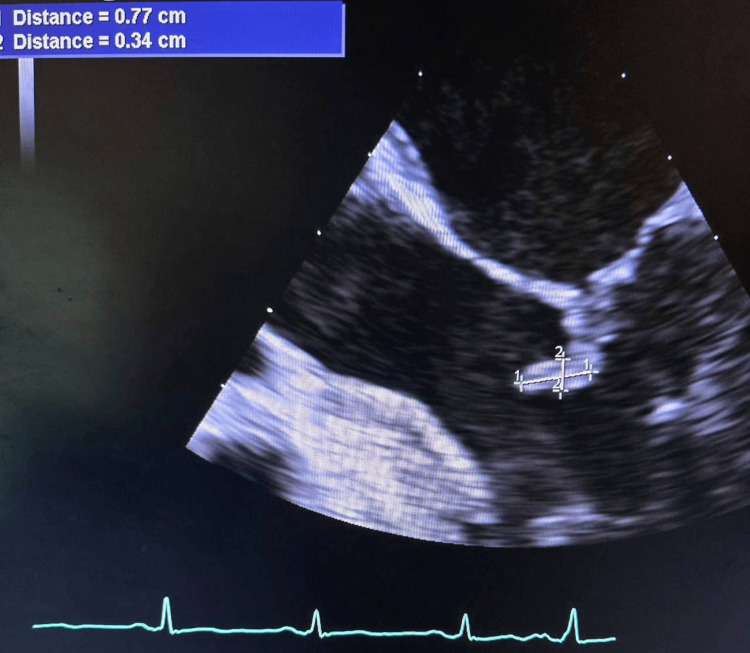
Echocardiographic image demonstrating mitral valve echodensity concerning for vegetation. Representative echocardiographic image demonstrating an echodensity attached to the mitral valve and concerning for vegetation. The lesion measured approximately 0.77 × 0.34 cm on echocardiographic assessment.
